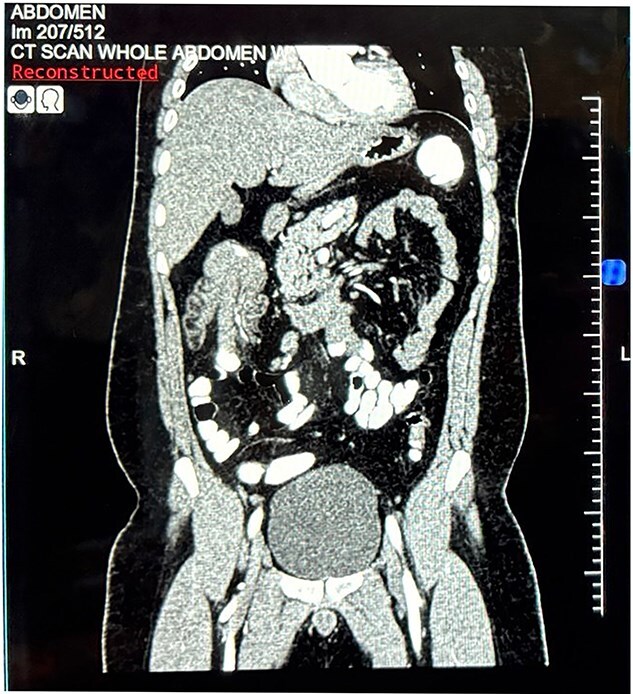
Coronal contrast-enhanced computed tomography of the abdomen. Coronal reconstruction demonstrating ileocolic intussusception extending to the hepatic flexure of the colon.
